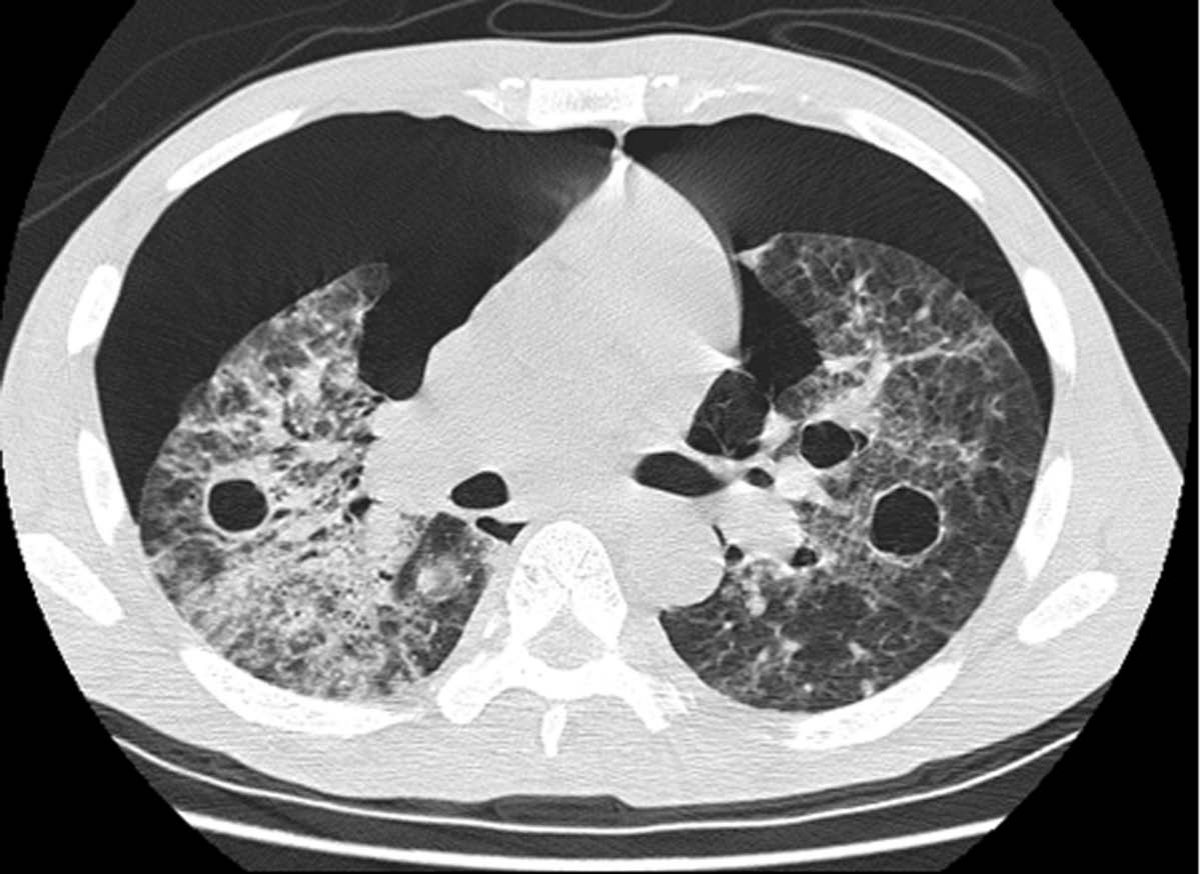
Chest computed tomography scan showing bilateral pneumothoraces, several pneumatoceles, bilateral airspace consolidation, and ground-glass opacity.
